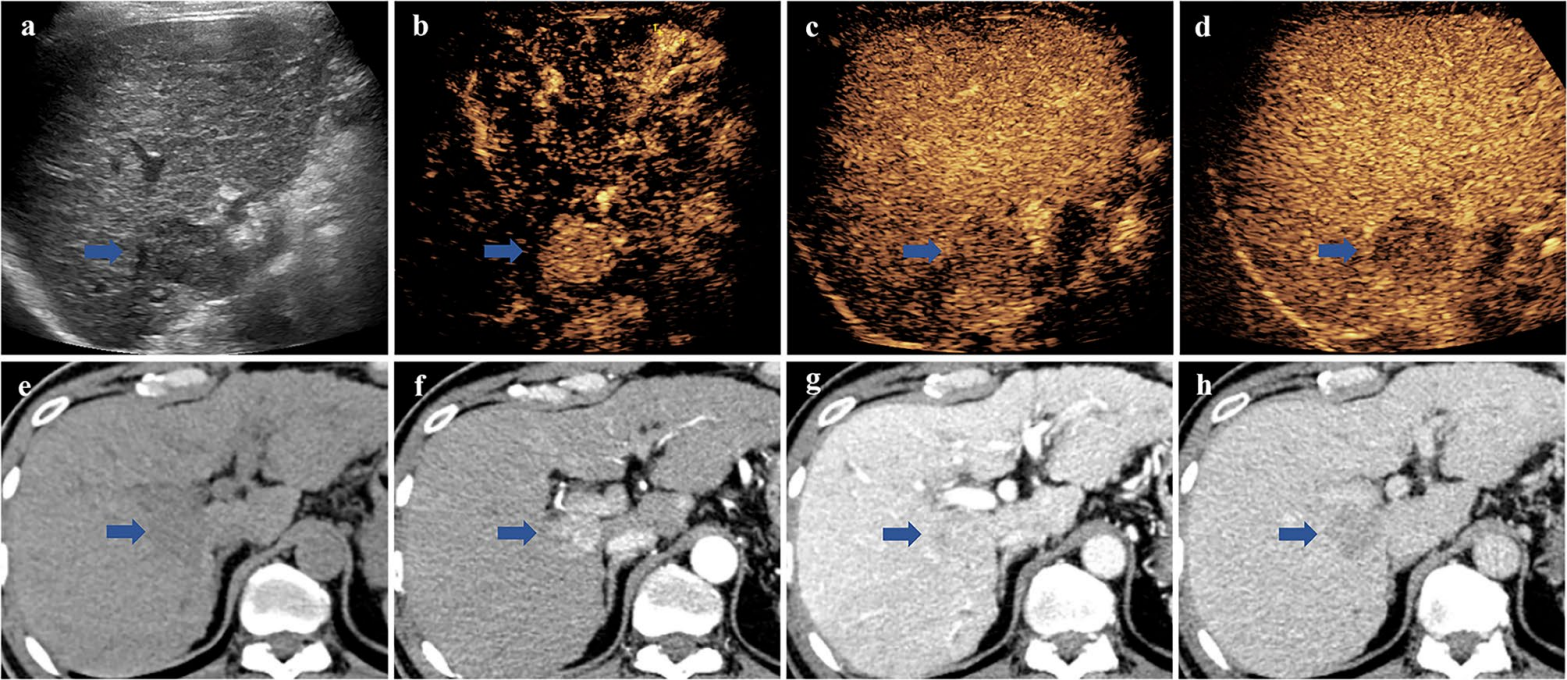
A lesion in segment 5 of the liver that was classified as LR-M via CEUS, LR-5 via CECT, and eventually pathologically confirmed as HCC in a man with hepatitis B-related cirrhosis. (a) Conventional ultrasound showed a hypoechoic mass measuring 3.2 × 2.1 cm in segment 5 of the liver. (b) CEUS displayed hyperenhancement in the arterial phase. (c) CEUS displayed early washout (42 s after injection) in the portal phase and (d) mild washout in the late phase. (e) Nonenhanced CT showed a hypodense mass measuring 2.9 × 2.8 cm in segment 5 of the liver. (f) CECT showed hyperenhancement in the arterial and portal (g) phases and washout in the late (h) phase with a visible enhancing capsule
